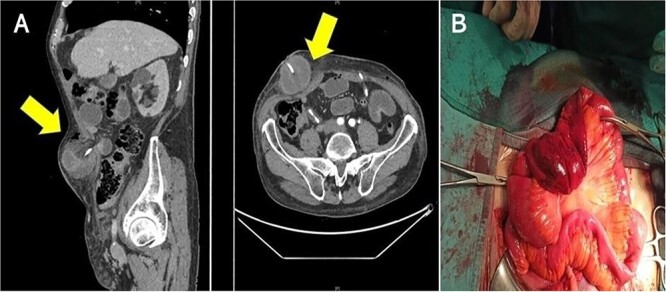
(A) Computed tomography shows the small intestinal herniation from the space between right ureter and trasversalis fascia; the arrows show hernial orifice; (B) surgeons observed engorgement of the bowel loops.
